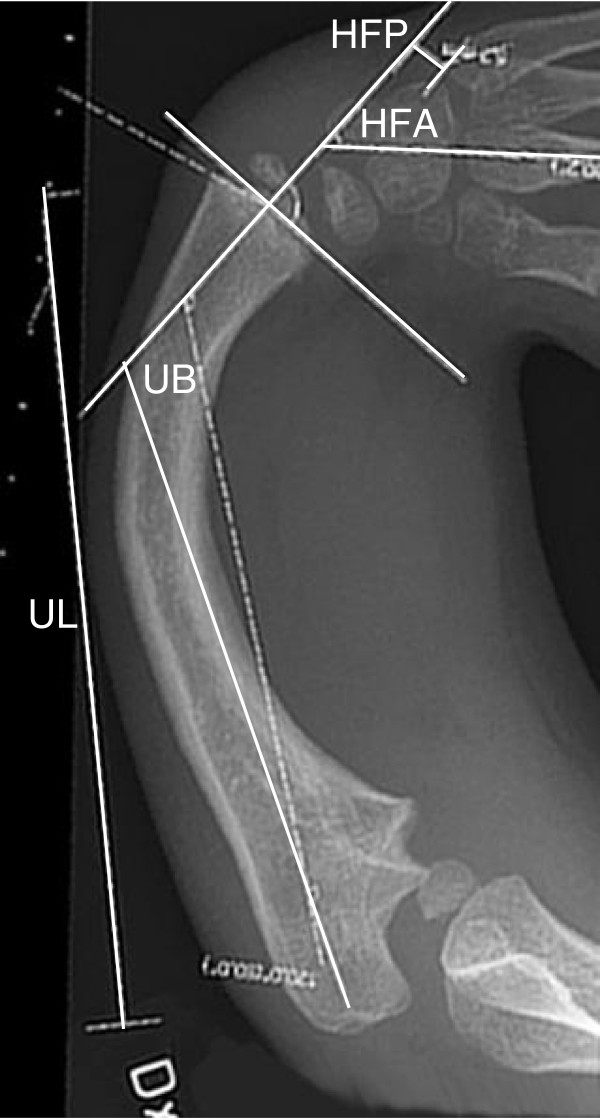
Radiographic measurements. Radiographic measurements were performed on the postero-anterior view of the forearm and hand. HFA (Hand Forearm Angle), HFP (Hand Forearm Position), UL (Ulnar Length), UB (Ulnar Bow).
